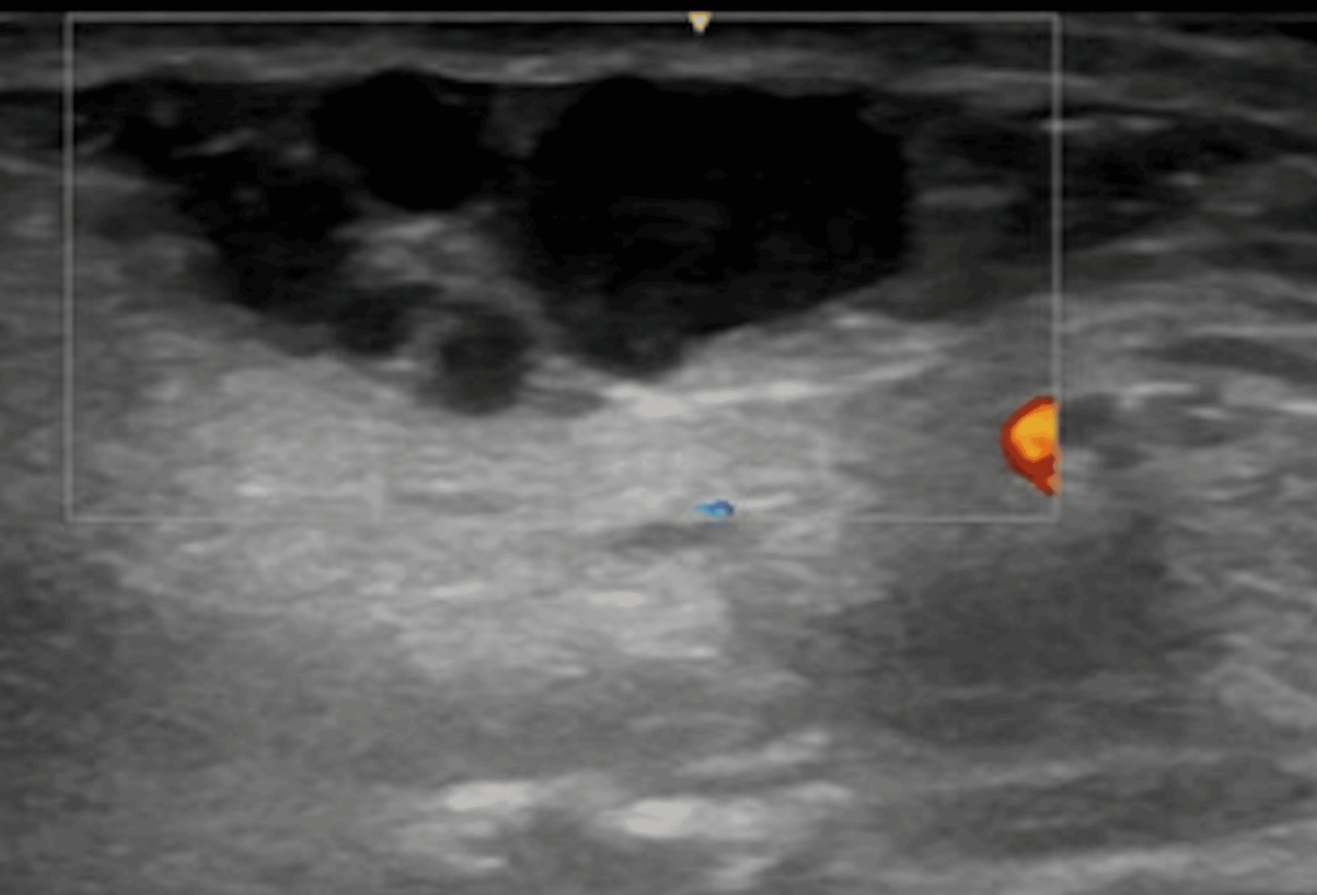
Ultrasound scan demonstrating lesions
